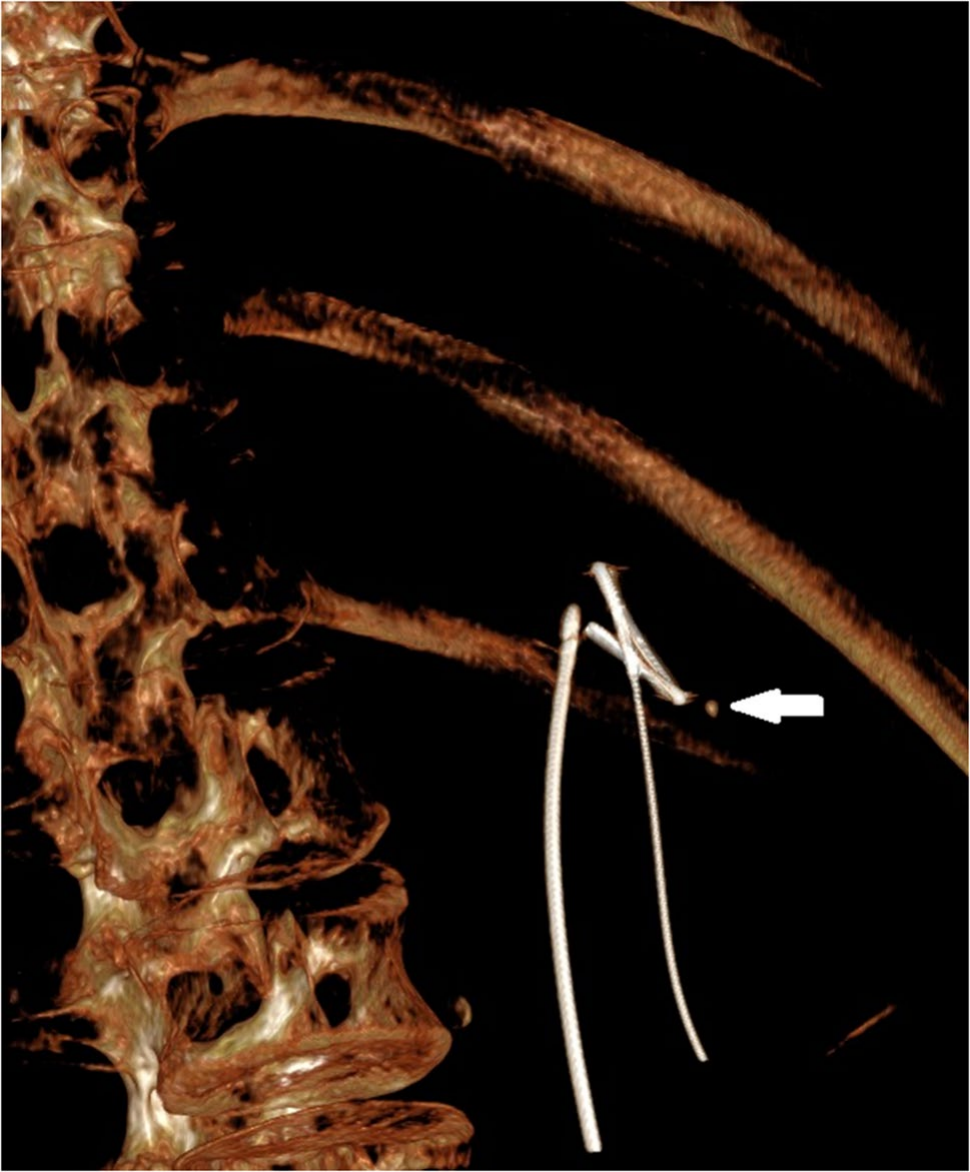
3D-reconstruction of the CBCT-scan in a patient with a residual fragment. The residual fragment is indicated by a white arrow. The straight wire represents an occlusion catheter. The wire with a loop represents the safety wire
